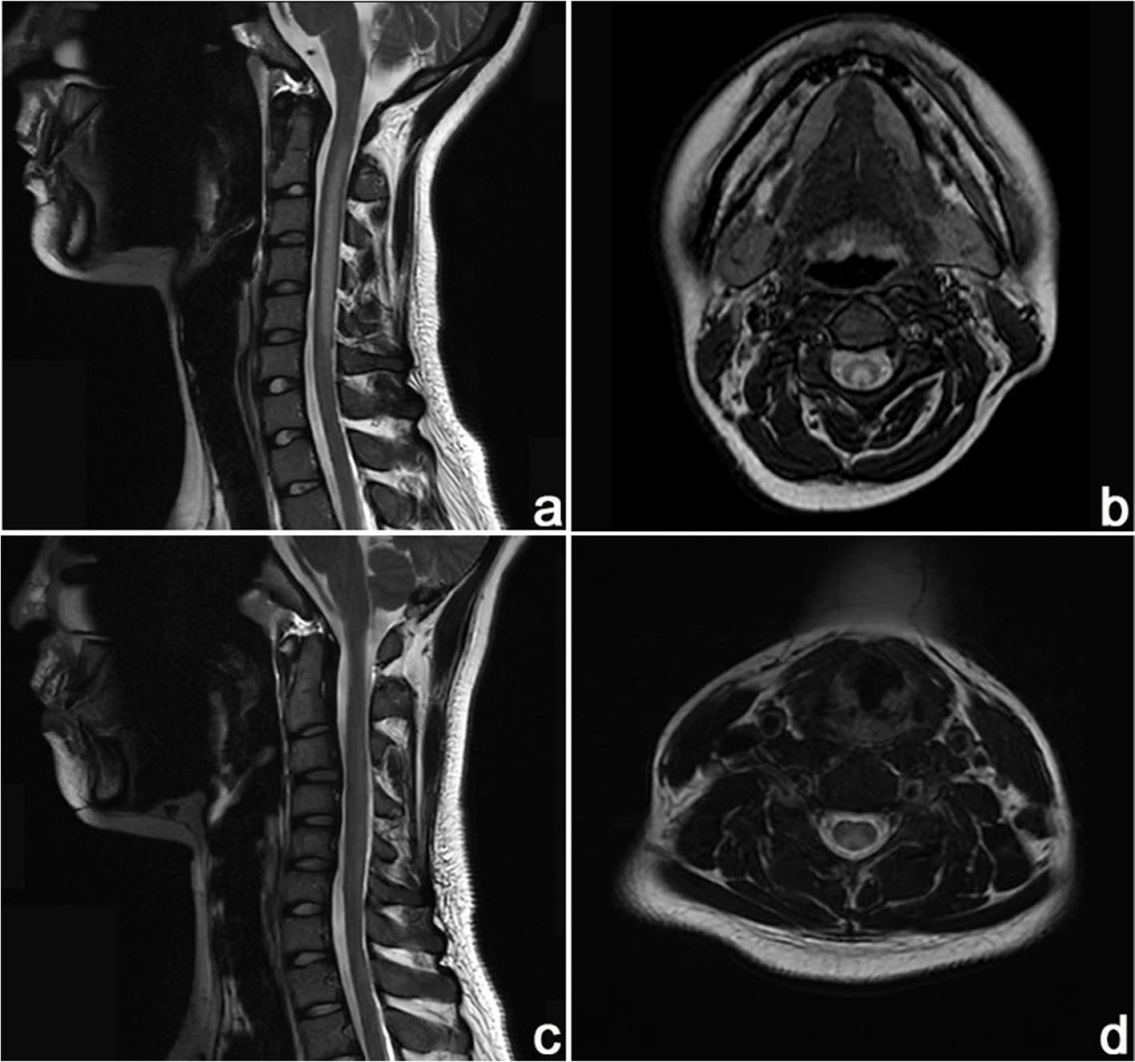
Spinal MRI findings of the two patients. In case 1, (a) sagittal T_2_-weighted imaging showed symmetrical hyperintensities from C2 to C5 segment in both posterior and lateral columns of the spinal cords, and (b) axial T2-weighted imaging revealed typical inverted “V”- shaped lesion. In case 2, (c) sagittal T_2_-weighted imaging showed symmetrical hyperintensities from C2 to T1 segment in both posterior and lateral columns of the spinal cords, and (d) axial T_2_-weighted imaging revealed typical inverted “V”- shaped lesion
